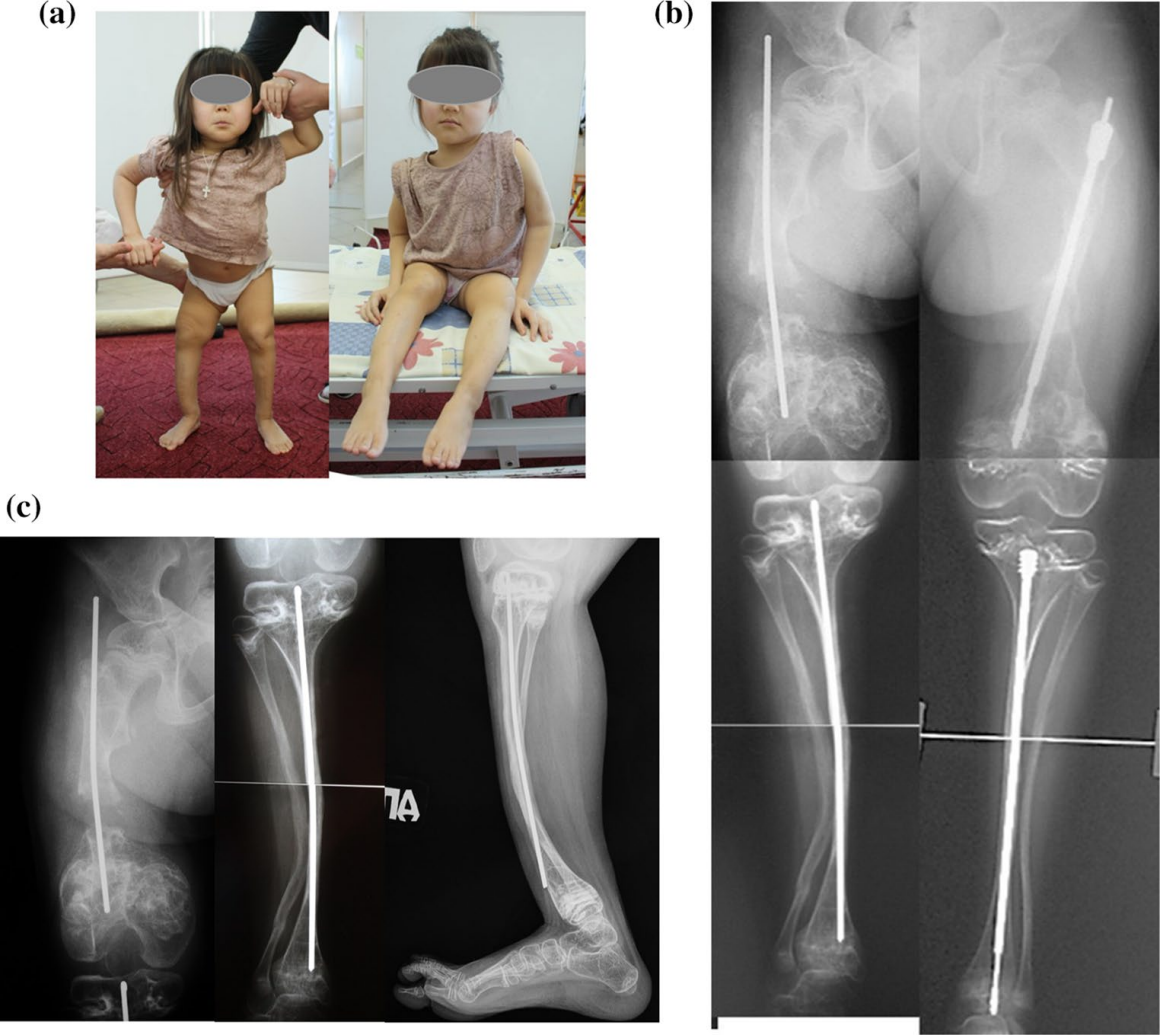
Girl, 9 years old with OI type VIII, before surgery: a patient before surgery; b standing anteroposterior lower limb radiographs; c bone defect and non-union of right femur after excessive bone resection (longitudinal instability of osteosynthesis with Rush rod), proximal migration of Rush rod, valgus-procurvatum deformity of right tibia with external migration of distal extremity of Rush rod through cortex
